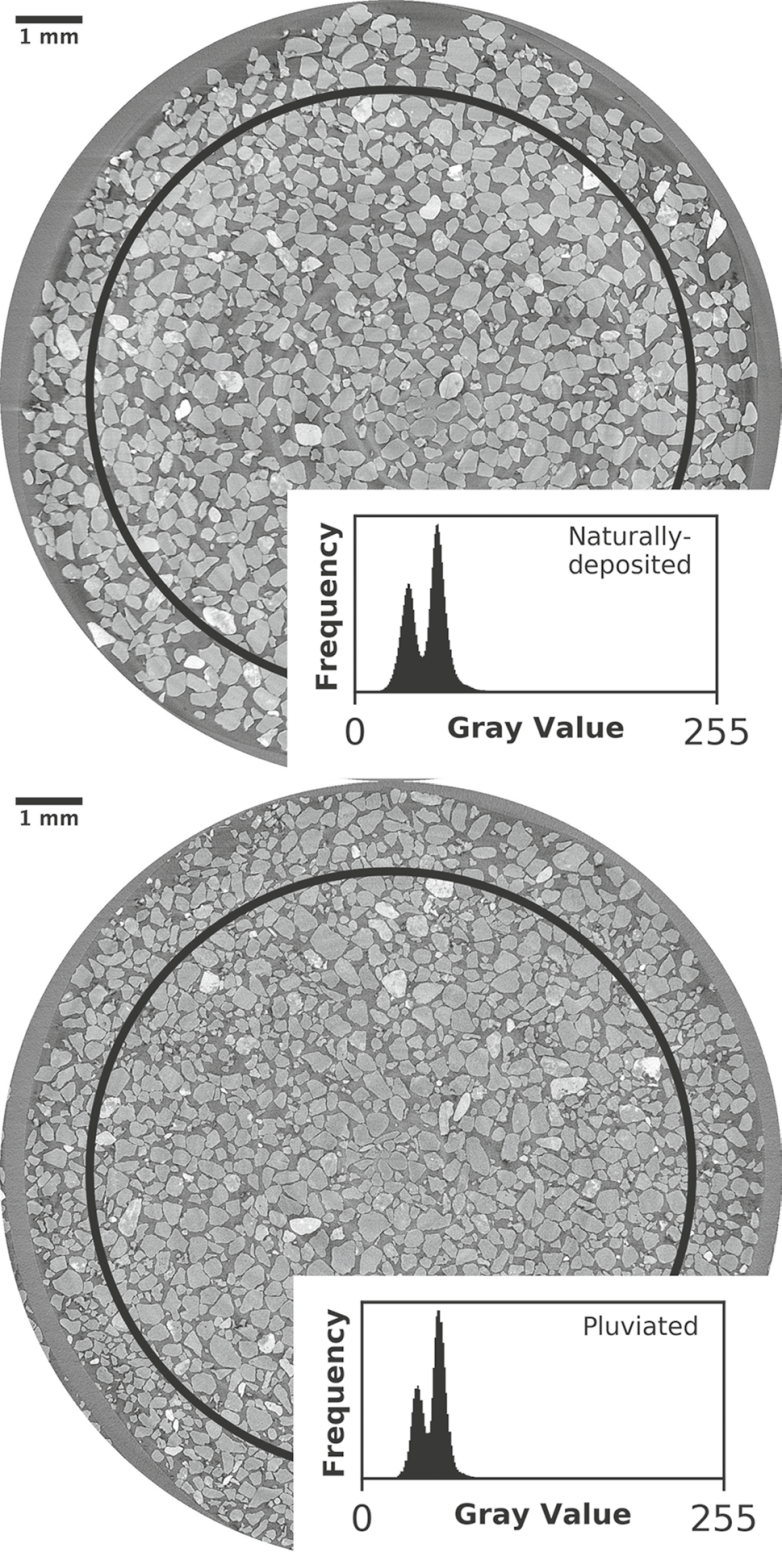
X-ray computed microtomography images showing horizontal cross-sections of the naturally-deposited (top) and pluviated (bottom) sands. The black circles denote the 9 mm diameter of the image subsection we consider in our analyses
